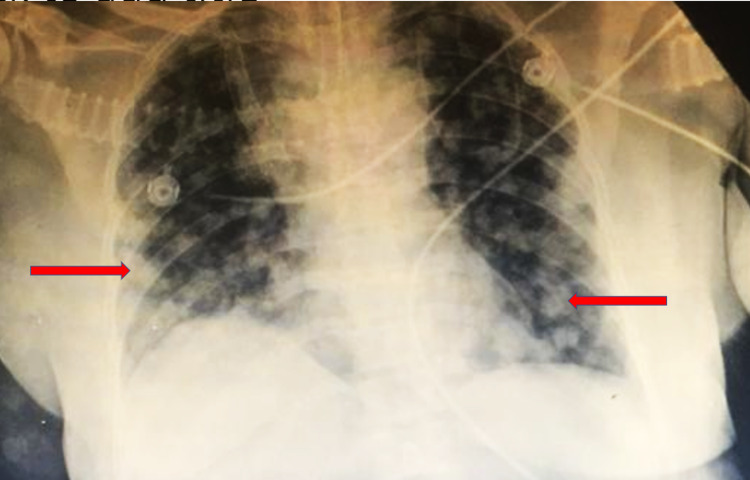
Patient’s chest X-ray on arrival showed multiple nodular opacities in bilateral lungs (as indicated with the red arrows).
